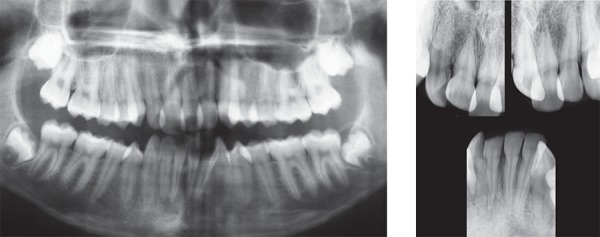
Initial panoramic and periapical radiographs of incisors.
